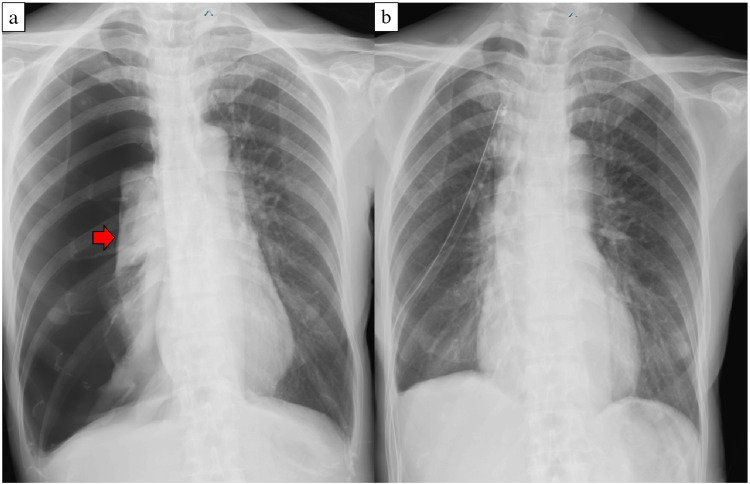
Chest radiographs at the time of initial examination a) The right lung is severely collapsed (red arrow). The mediastinum is displaced to the left, and a diaphragmatic depression is observed; b) After insertion of the right chest tube. The expansion of the right lung improved.
